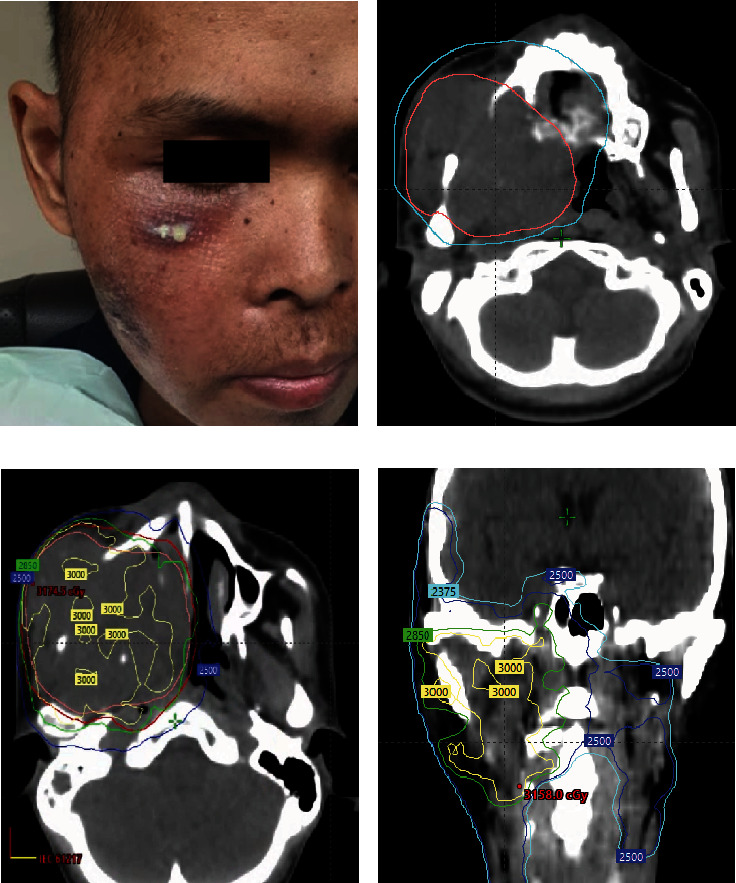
Two weeks posttreatment evaluation and second radiotherapy of 30 Gy in five fractions delivered every other day. (a) Clinical picture of the patient before the second treatment reveals excellent clinical response. (b) Axial view of CT scans showing a reduction in the size of the initial tumor (cyan) and current tumor (pink). (c) Axial view of the second radiotherapy plan. (d) Coronal view of the second radiotherapy plan.
